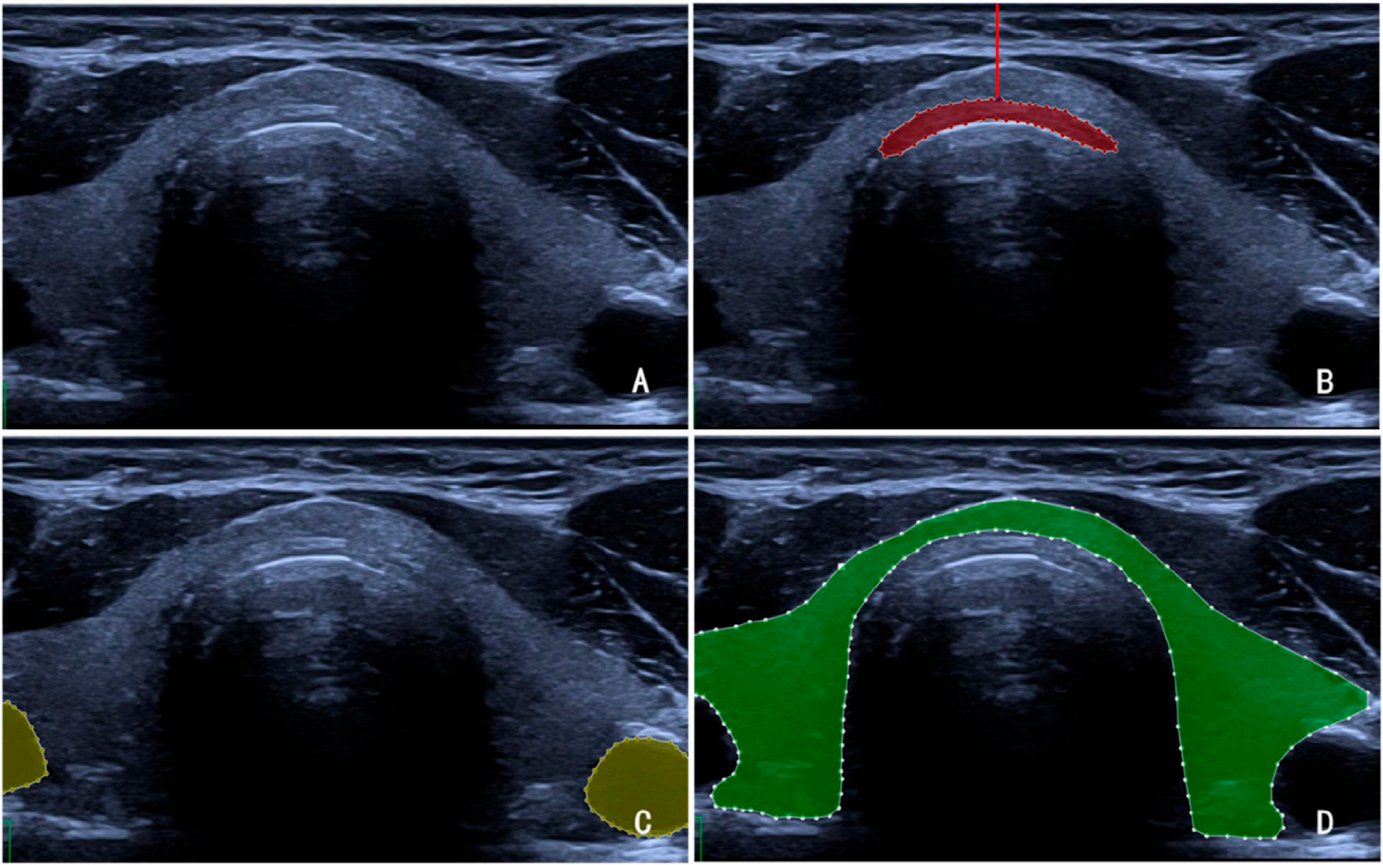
(A) Ultrasound image of a cadaver’s neck. (B) The red-shaded region in the figure denotes the tracheal rings, while the red line indicates the tracheal depth at that site, defined as the vertical distance from the trachea to the cervical skin surface. (C) The yellow-shaded region represents the vascular structures. (D) The green-shaded region represents the thyroid gland.
